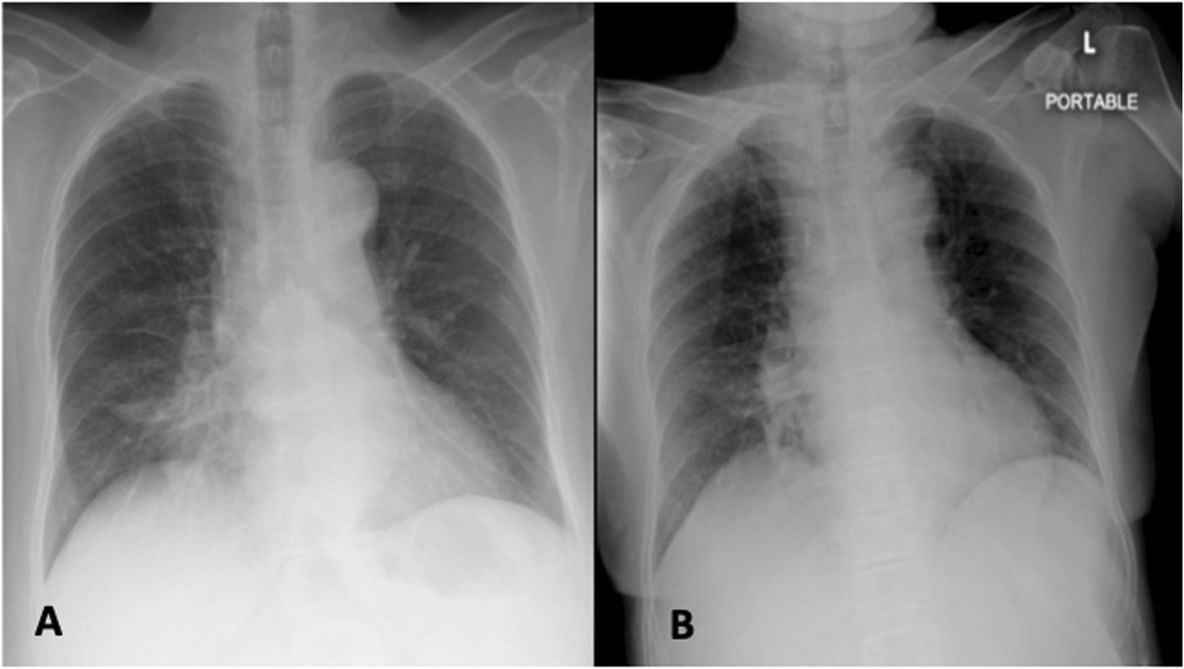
Chest radiograph 3 months prior to admission showing right middle lobe pneumonia (a) and on admission showing minimal infiltration at medial aspect of right lower lung (b)
